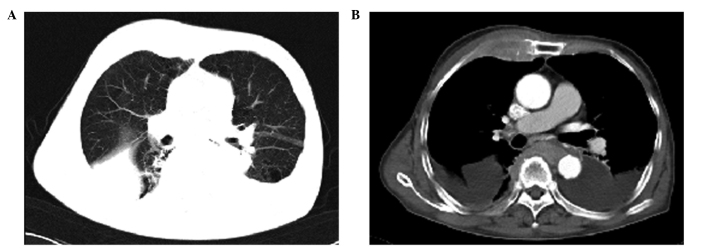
Computed tomography scans showing bilateral pleural effusion with no evident mass lesions in the (A) lungs and (B) mediastinal windows.
